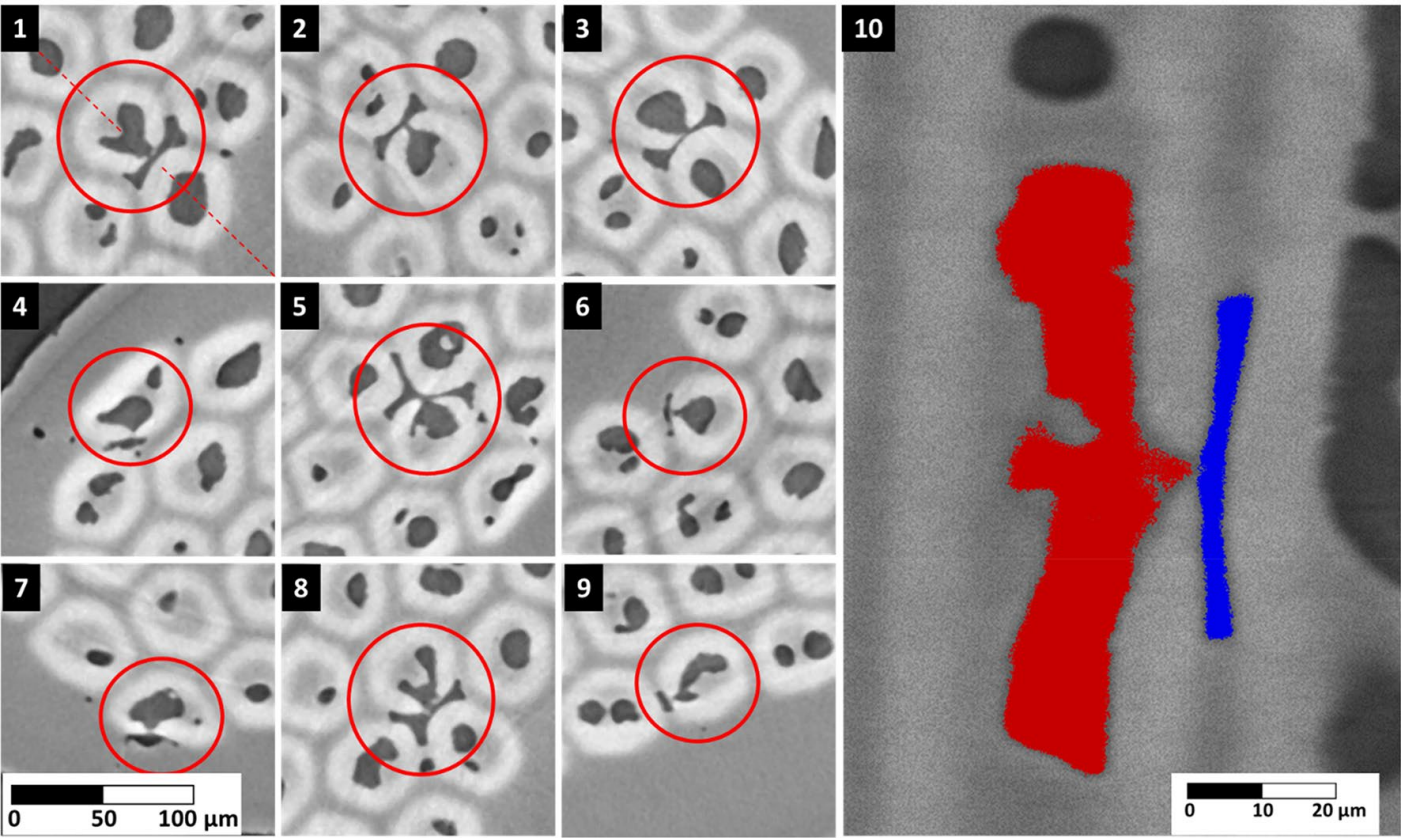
Collection of Cu voids generated by Sn diffusion due to damaged Nb barriers in S20. The void in the sub-element is connected to the Cu void crossing the Nb_3_Sn ring at the Nb barrier rupture. Pictures 1 to 9 are transversal cross sections of the wire. Picture 10 shows the longitudinal cross section of void #1 cut along the red dashed diagonal shown in picture 1. The void is highlighted in red inside the sub-element and in blue in the Cu matrix. The images are obtained from the 3D reconstruction of the X-ray tomographies.
